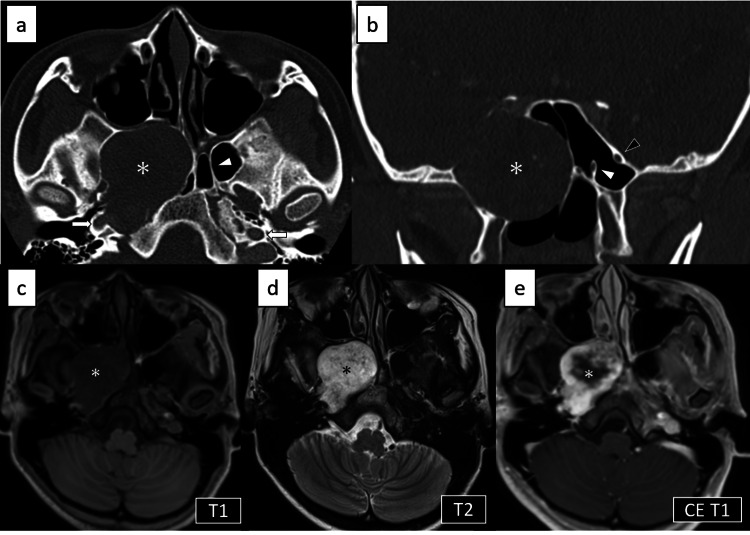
Preoperative CT and MRI The tumor occupied the floor of the sphenoid sinus and displaced the pterygopalatine fossa anteriorly on the right side (asterisk). a) Axial plain CT image. Vidian canal and foramen lacerum on the right side were not detected. White arrowhead: left vidian canal. White arrows: carotid canals. b) Coronal plain CT image. The surrounding bone was thinned, but no bony invasion was present. We were able to identify the vidian canal and foramen rotundum on the left side but not on the right side. White arrowhead: left vidian canal. Black arrowhead: left foramen rotundum. On MRI, the tumor was (c) low signal on T1-weighted image, (d) high signal on T2-weighted image, and (e) slightly and heterogeneously enhanced.
